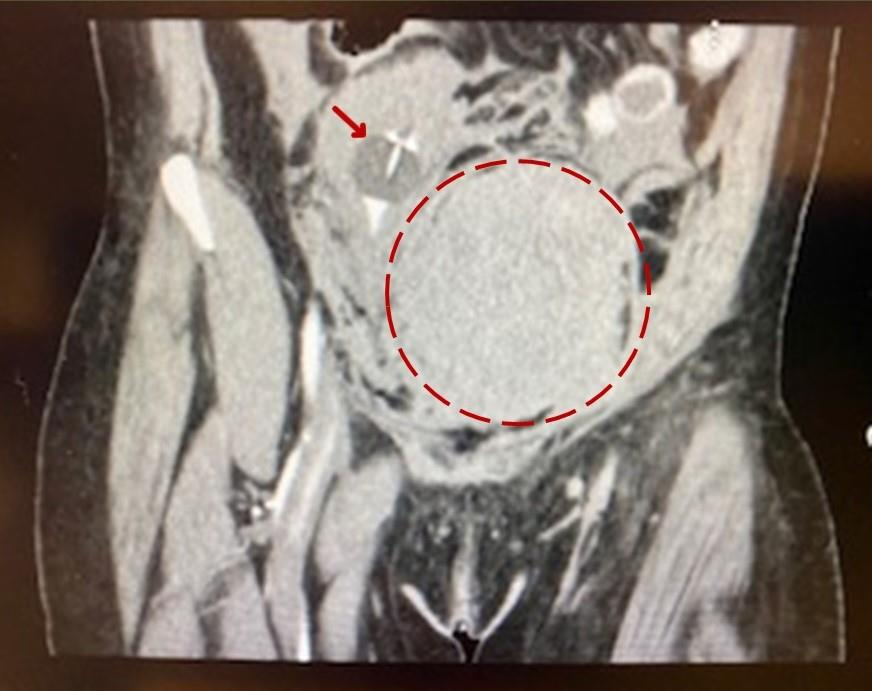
Repeat computed tomography of the abdomen and pelvis three hours later, with significantly expanded hematoma. Foley in compressed urinary bladder and bladder displaced. *Red arrow*: Foley in compressed urinary bladder and bladder displaced. *Dashed circle*: expanded pelvic hematoma.
